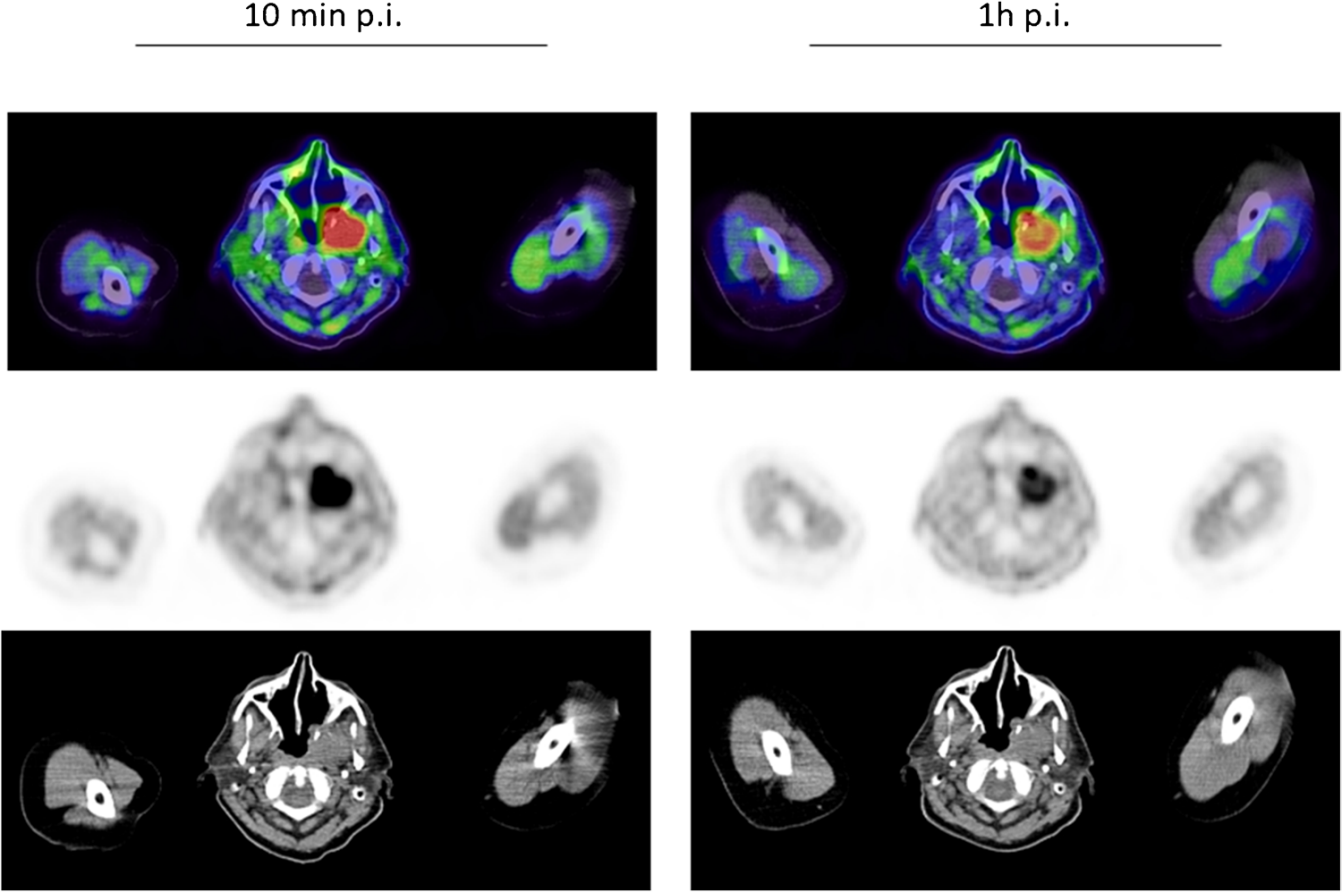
A 77-year-old patient with a retro maxillary located metastasized esthesioneuroblastoma underwent ^68^ Ga-FAPI-PET/CT due to restaging. Remarkably, the quantified tracer uptake was higher 10 min after tracer application with a SUVmax of 10.75 than 1 h p.i. with a SUVmax of 7.69, respectively
